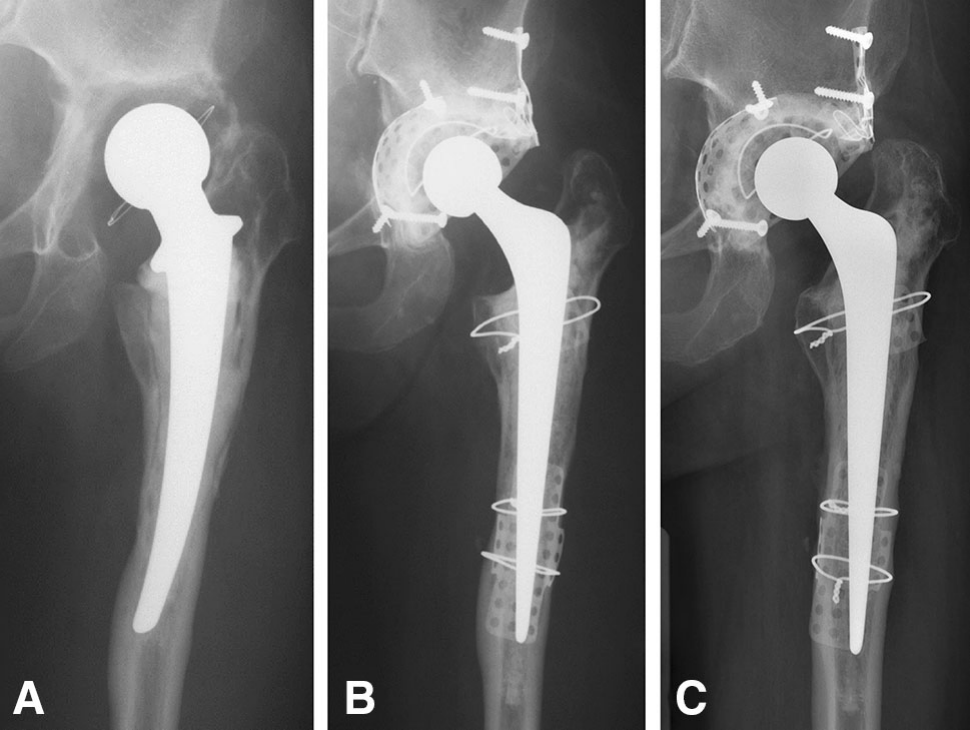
(A) Preoperative radiograph of a female patient, about to undergo THA, showing aseptic loosening and bone loss of both the acetabulum and proximal femur. The patient had previously undergone total cemented revision implantation. The revision components were in situ for 11 years. The patient was 19 years old when the primary total hip prosthesis was implanted after a coxitis. The primary THA was revised after 15 years. (B) Radiograph after the index revision surgery with IBG of both the acetabulum and the femur. The patient was 45 years old at the time of the revision. Segmental bone defects of the medial and lateral acetabular wall were reconstructed with metal meshes and screws. On the femoral side, a distal cortical perforation was covered with a metal mesh and cerclage wires, and the dorsal calcar region was also reconstructed. (C) Radiograph at final followup 19 years after the index revision with IBG. Both revision implants are stable with incorporation of the bone grafts, although a small osteolytic area can be seen in Gruen Zone 1 of the femur. The patient was 64 years old at last followup and still has an excellent functioning hip (HHS 95) 45 years after the first THA.
